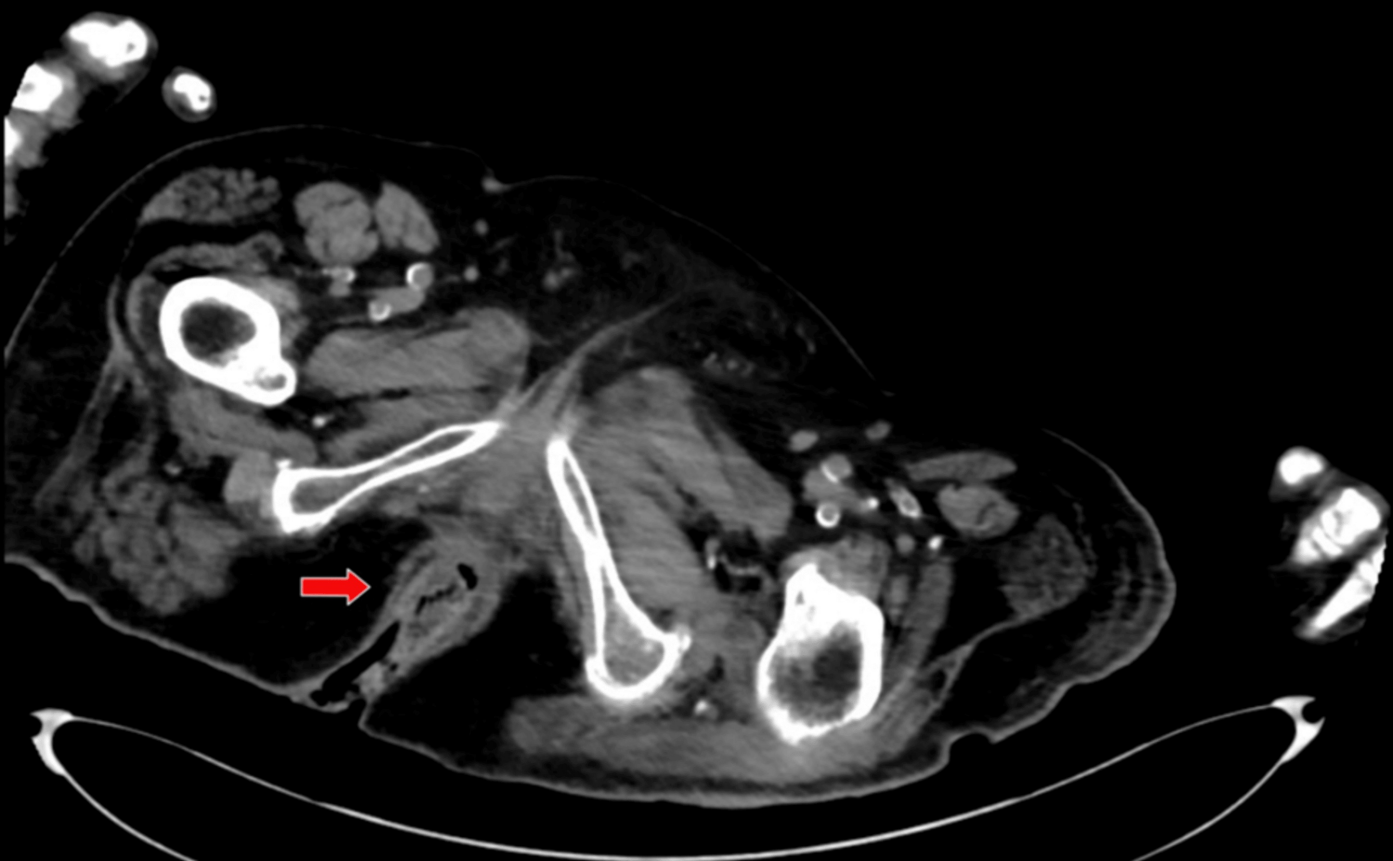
CT abdominal/pelvis view of stercoral colitis showing rectal wall thickening and inflammation
